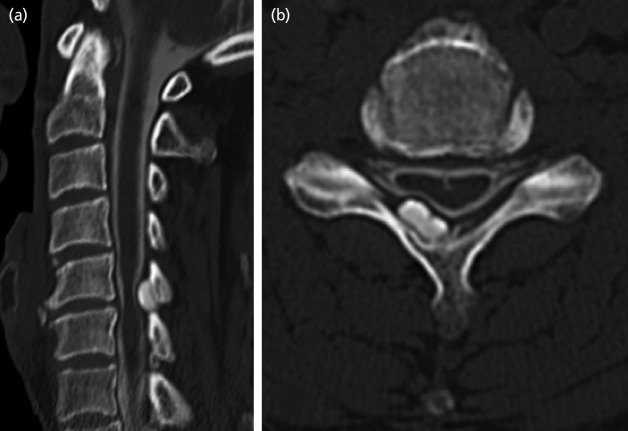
Case 4. CT after myelography showing an osseous lesion in continuity with the lamina and protruding into the spinal canal, with compression of the dural sac. (a) sagittal image, (b) axial images.
